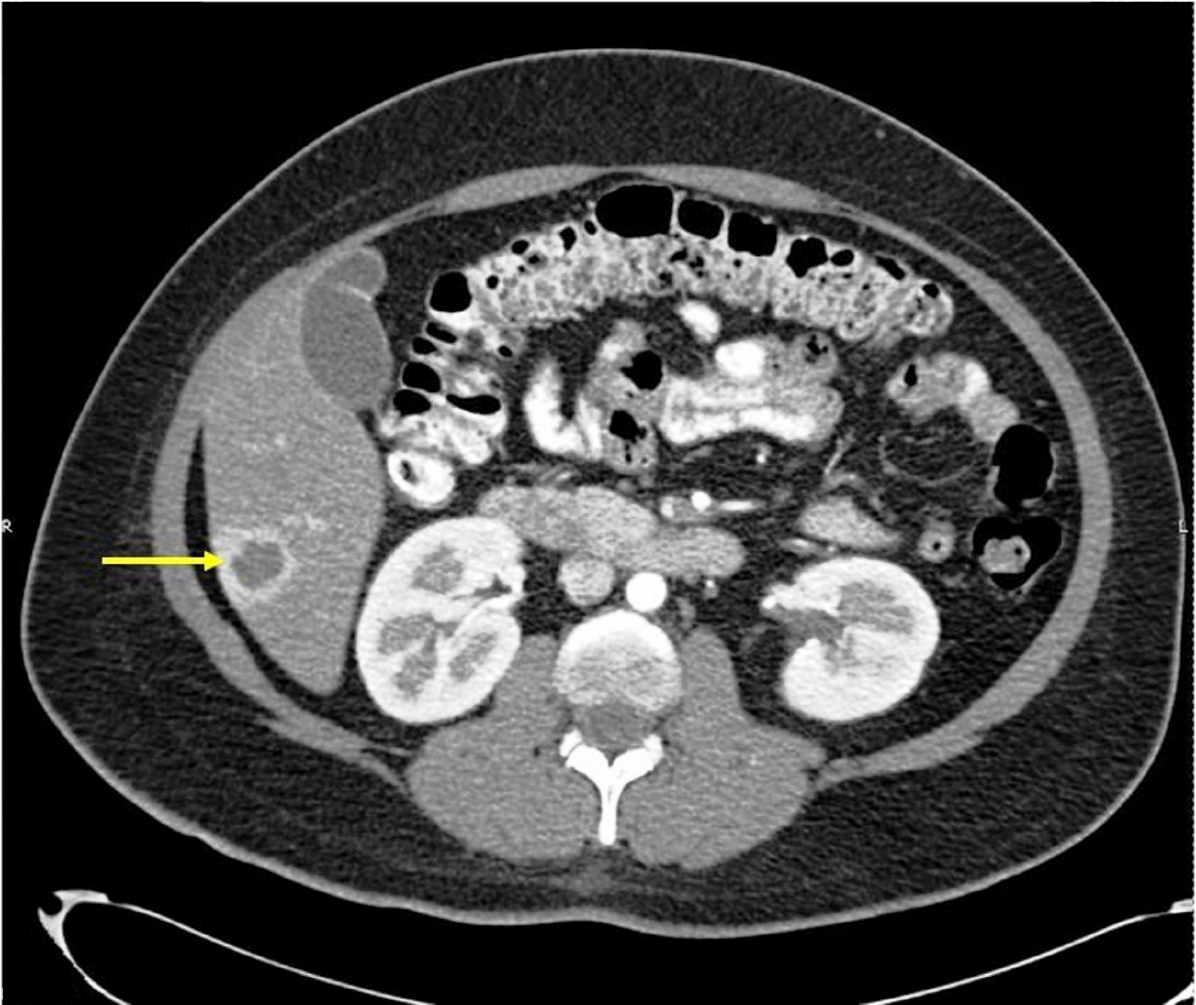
CT of the abdomen with contrast in venous phase The hepatic lesion became progressively isodense in venous phase.
